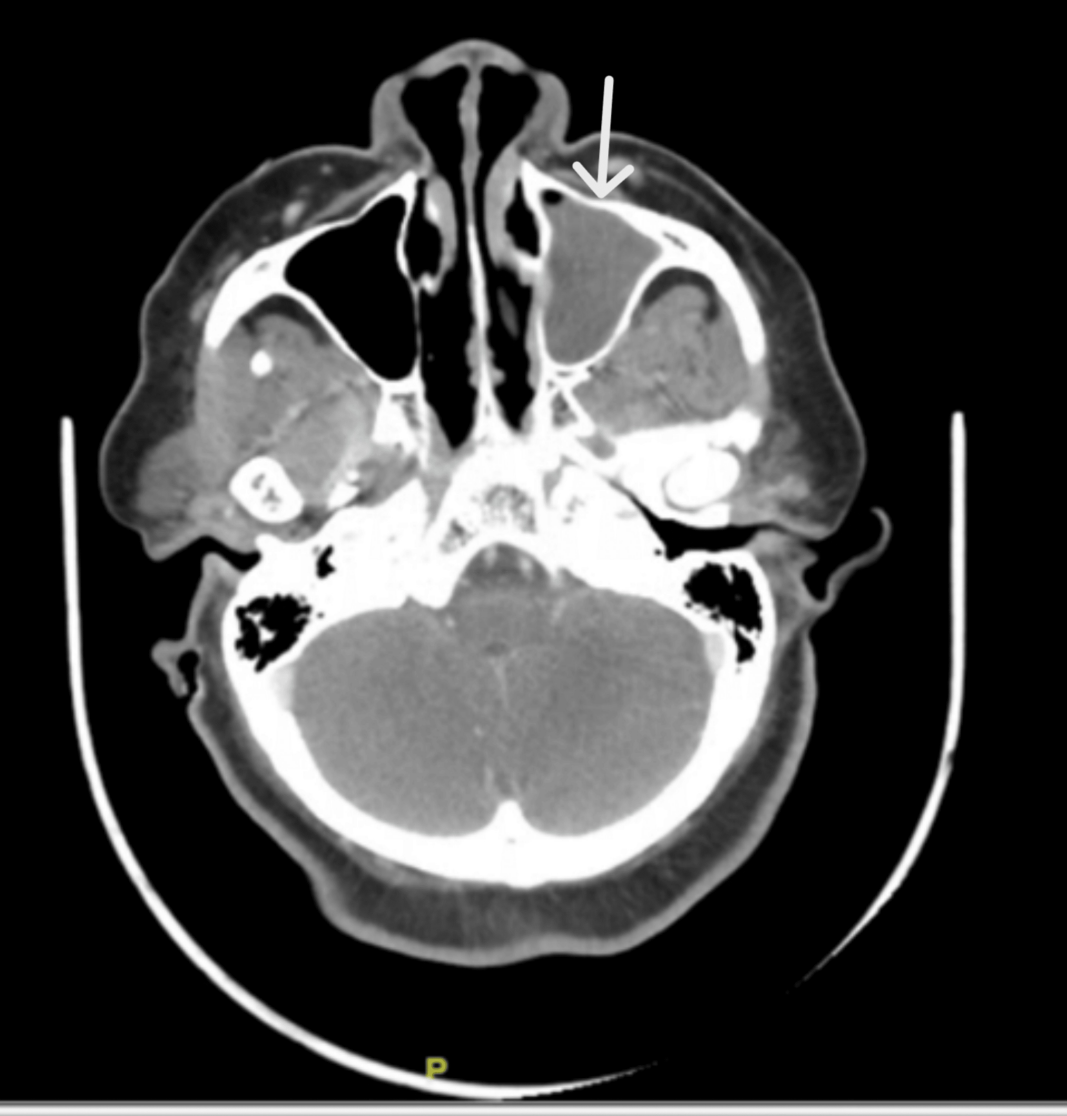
The axial section of the head CT showing opacification of the left maxillary sinus (marked by the white arrow)
